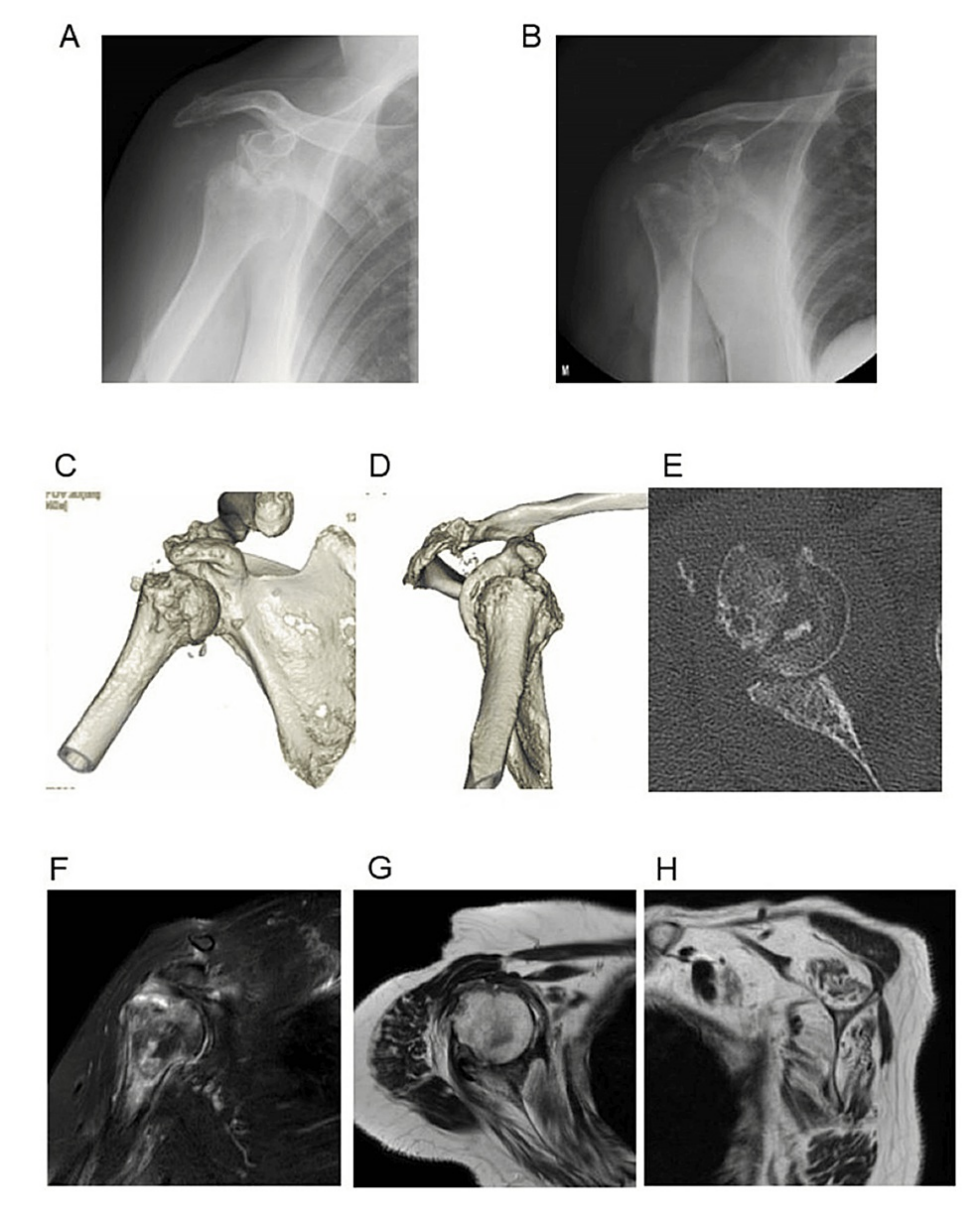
The preoperative images. A) Shoulder X-ray at the initial visit to our hospital. B) X-ray fluoroscopy after the attempted closed reduction. C-E) CT scans showing a large Hill-Sachs lesion and defect of the anterior glenoid surface. F) T2-weighted fat suppression image on coronal MRI showing a broad bone bruise in the humeral head. G, H) T2-weighted MRI showing severe muscle atrophy and fatty infiltration of the subscapularis, supraspinatus, and infraspinatus, indicating chronic significant rotator cuff tear.
